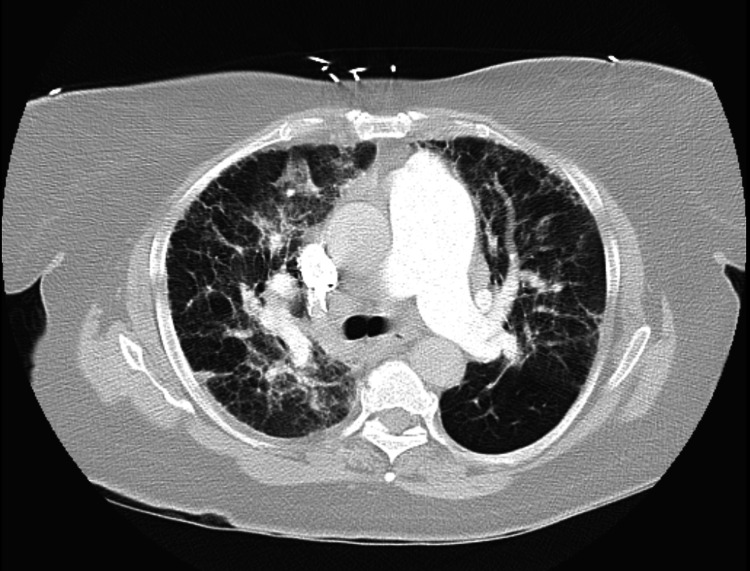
CT chest axial view lung window showed diffuse bilateral multifocal ground-glass opacities with prominent interlobular septa in the lower lobes.
